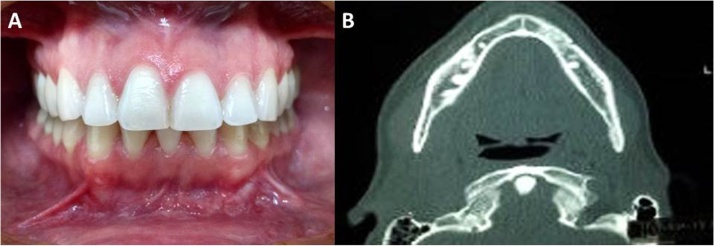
A – After 12 months of clinical control, bone repair and the absence of signs of mandibular lesion were detected at the site. B – Axial cut of the postoperative computed tomography, with discrete change in the trabecular bone in the mandible.
